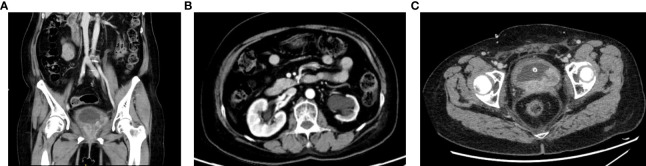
(A–C) Abdominal computed tomography (January 6, 2022).
